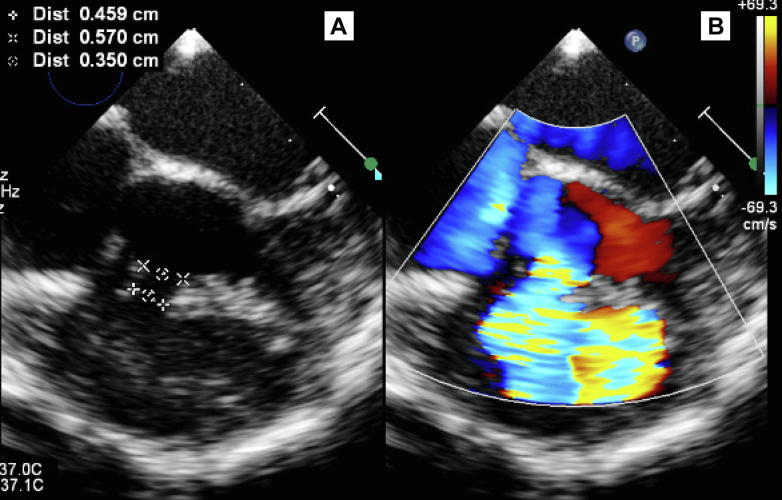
Transesophageal Echocardiography (Mid-Esophageal View, 0°) (A) Two-dimensional measurements showing defect size (left ventricular entry 5.7 mm, right ventricular exit 4.6 mm, length 3.5 mm). (B) Color Doppler demonstrating a left-to-right shunt.
